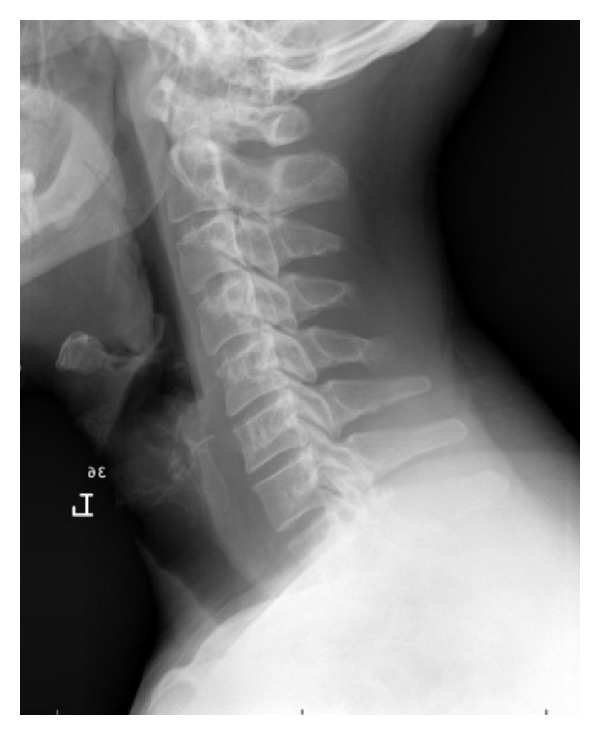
Lateral radiograph of the cervical spine in a patient who underwent laminoplasty. Note that there is an overall lordotic alignment which will allow for posterior drift once a posterior decompression is performed.
